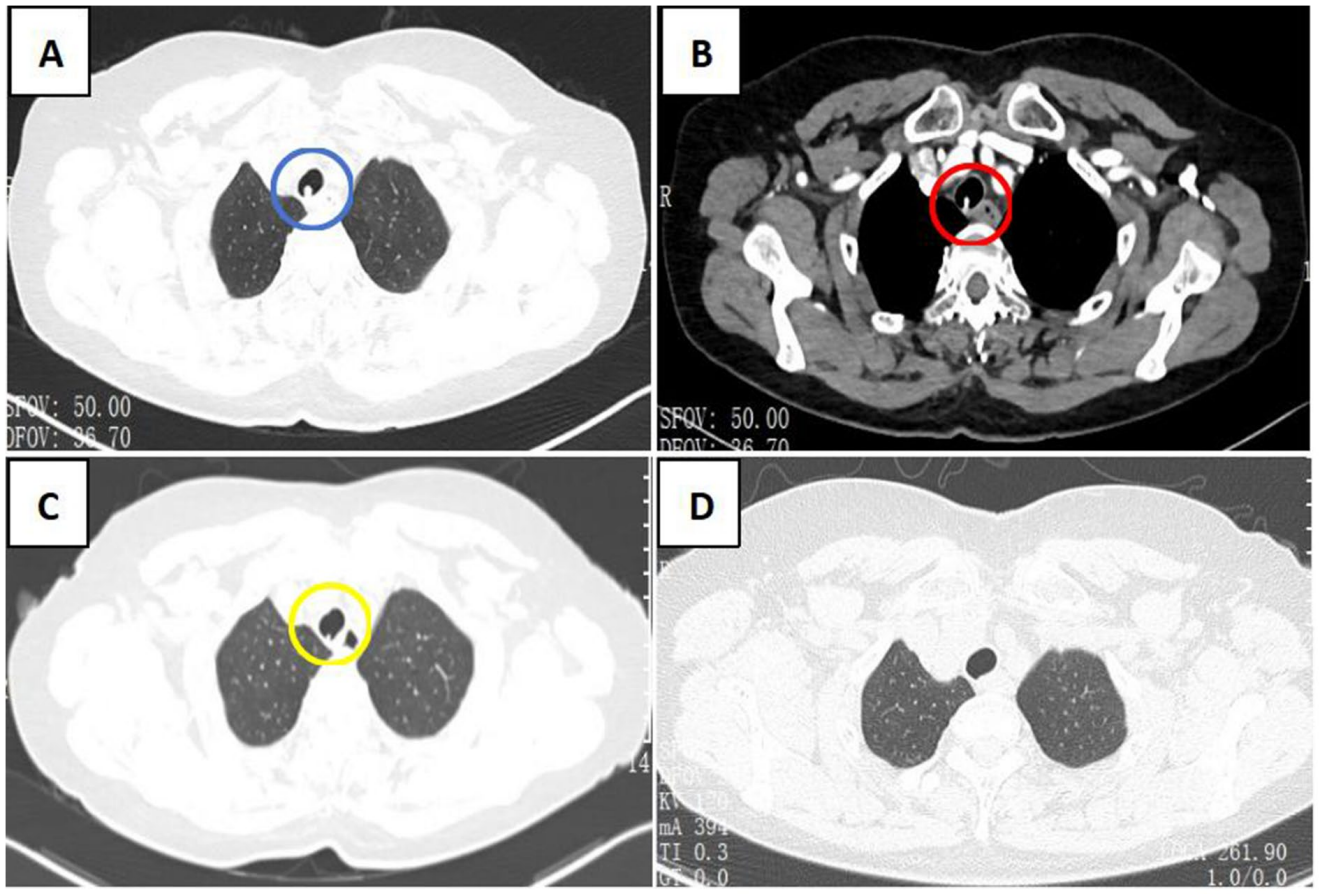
CT scan of the chest. (A) CT scan of the chest shows a new spherical organism in the upper tracheal segment with a smooth surface (blue circle). (B) CTA scan of the chest shows enhanced nodules in the tracheal, which originated from the branches of the thyrocervical trunk (red circle). (C,D) The organism shrank significantly after 1 month (yellow circle) and 4 months, respectively.
